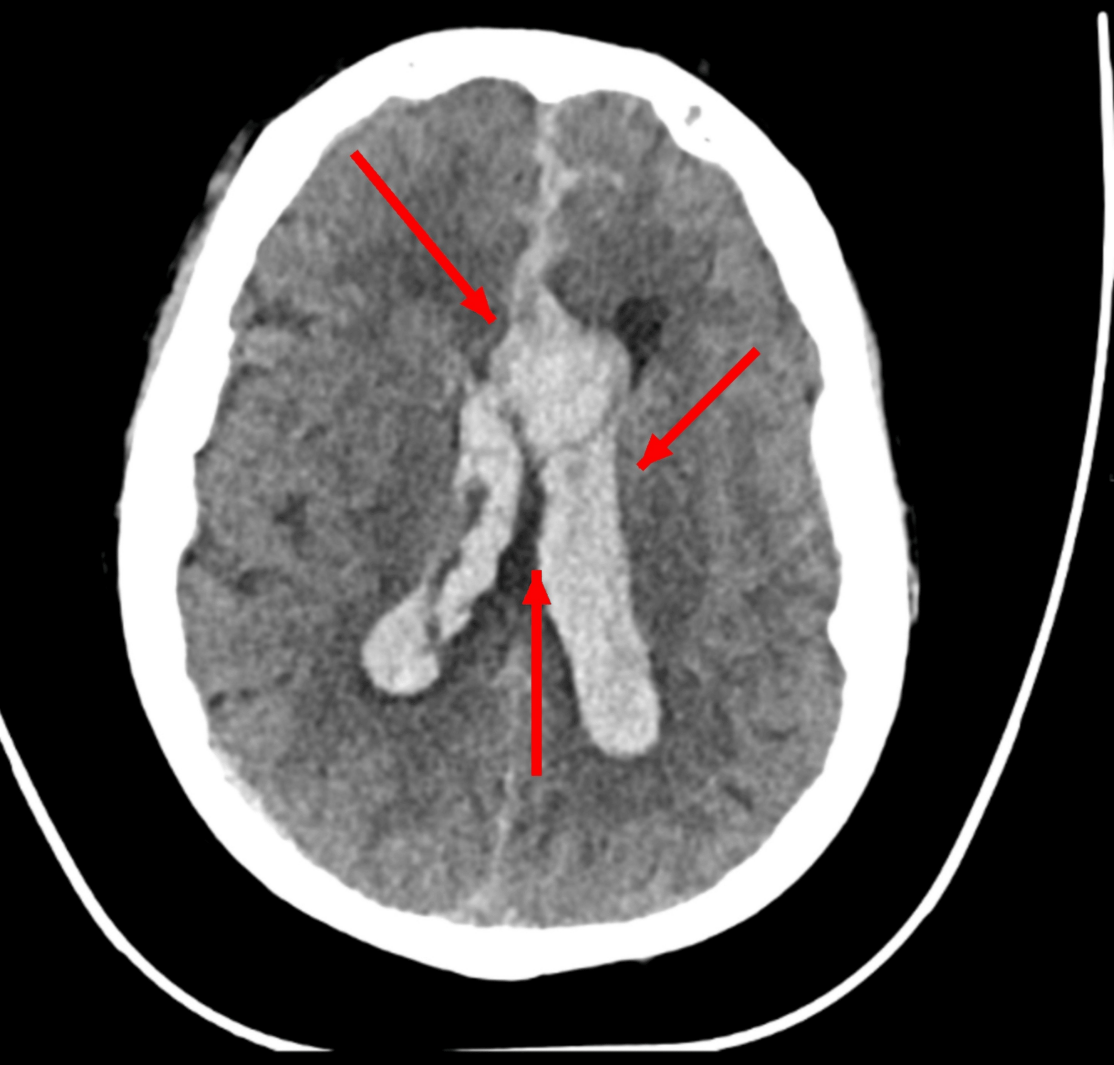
Axial section CT scan at the level of the lateral ventricle showing intra-parenchymal, subarachnoid, and intraventricular bleeding (CT scan at presentation).
